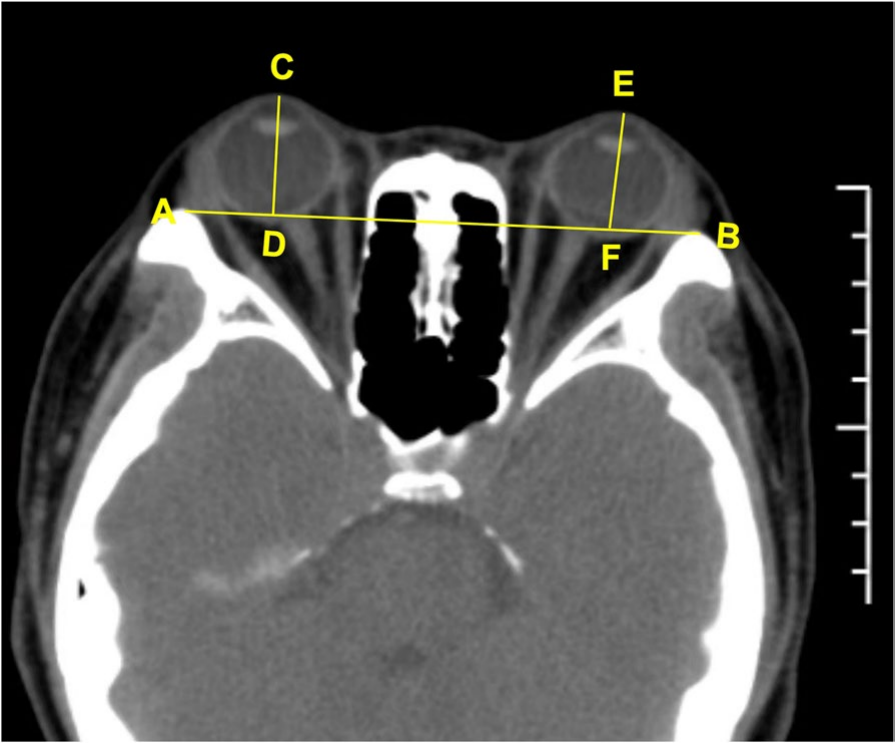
The measure exophthalmos based on CT imaging. AB is the orbital outer edge connection line, CD is the vertical distance from the corneal apex to the connection line of the right eye, which is the ocular protrusion. EF is the ocular protrusion of the left eye
